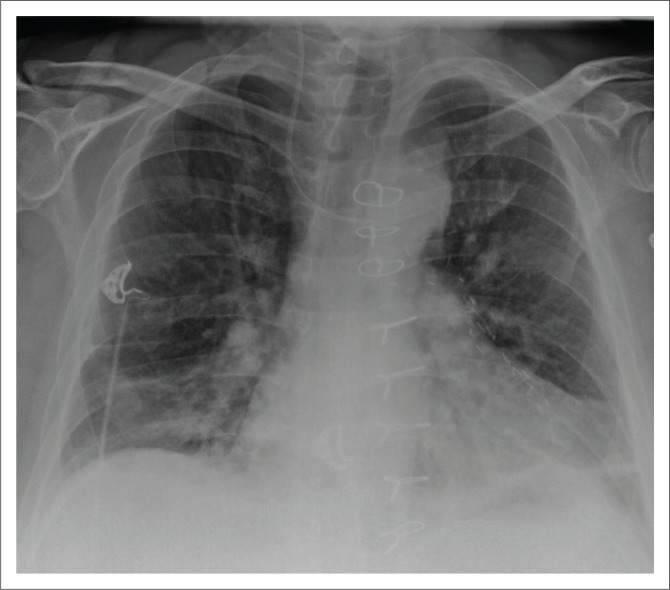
Magnified chest radiograph showing a malpositioned right central venous catheter in the left brachiocephalic vein.
